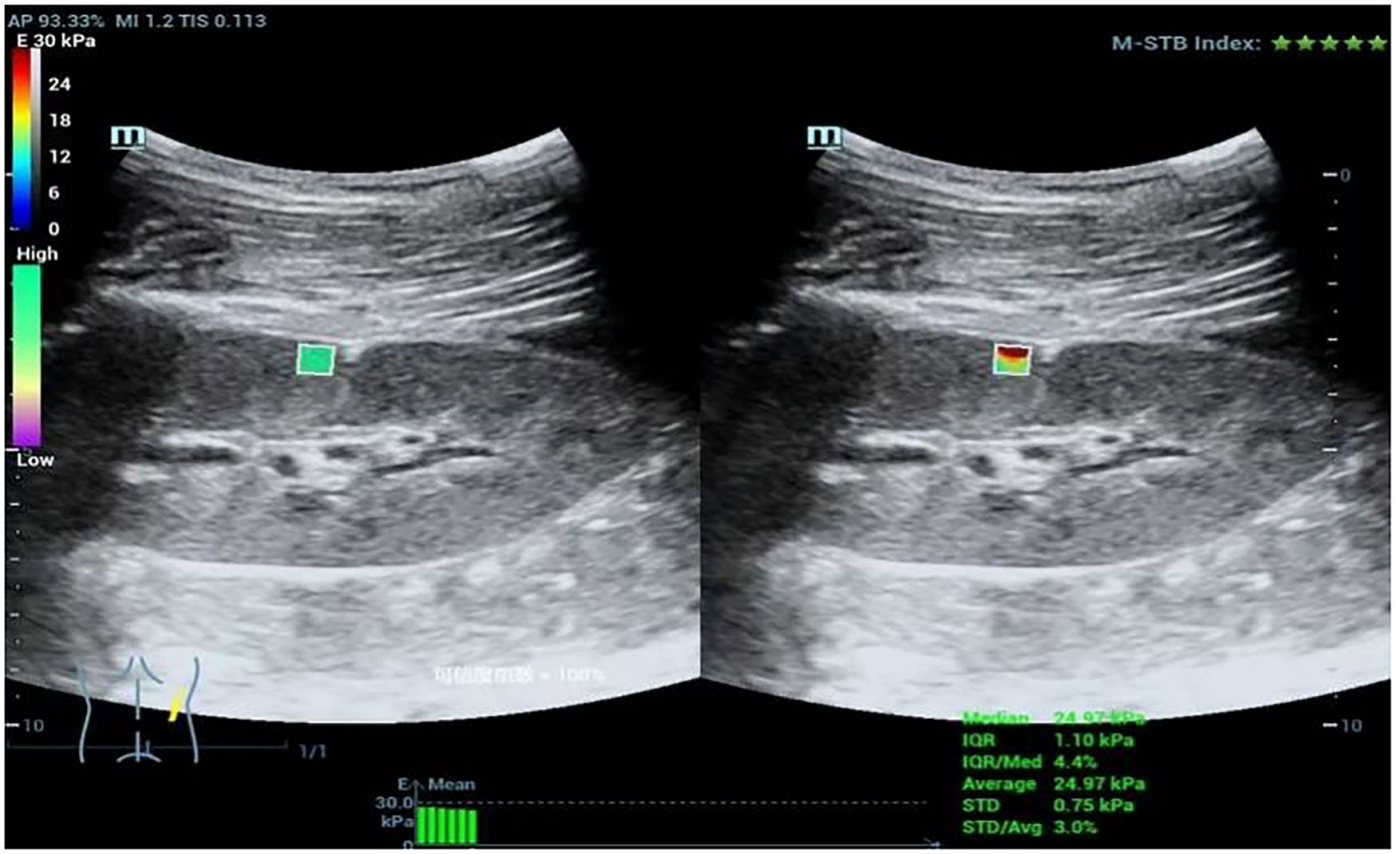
Ultrasound elastography in the PNS patients
